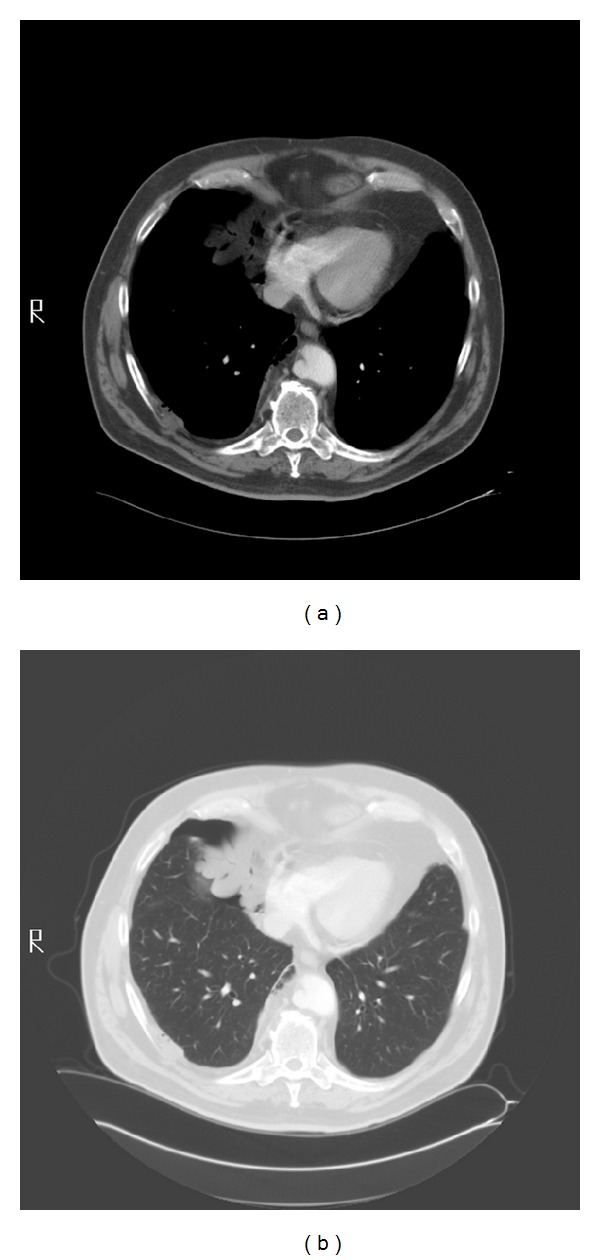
Chest CT scan in December 2007 showing a slightly larger anterior right pneumothorax. Corresponding soft tissue (mediastinal window view) showing that the soft-tissue mass adjacent to the pneumothorax is fat.
